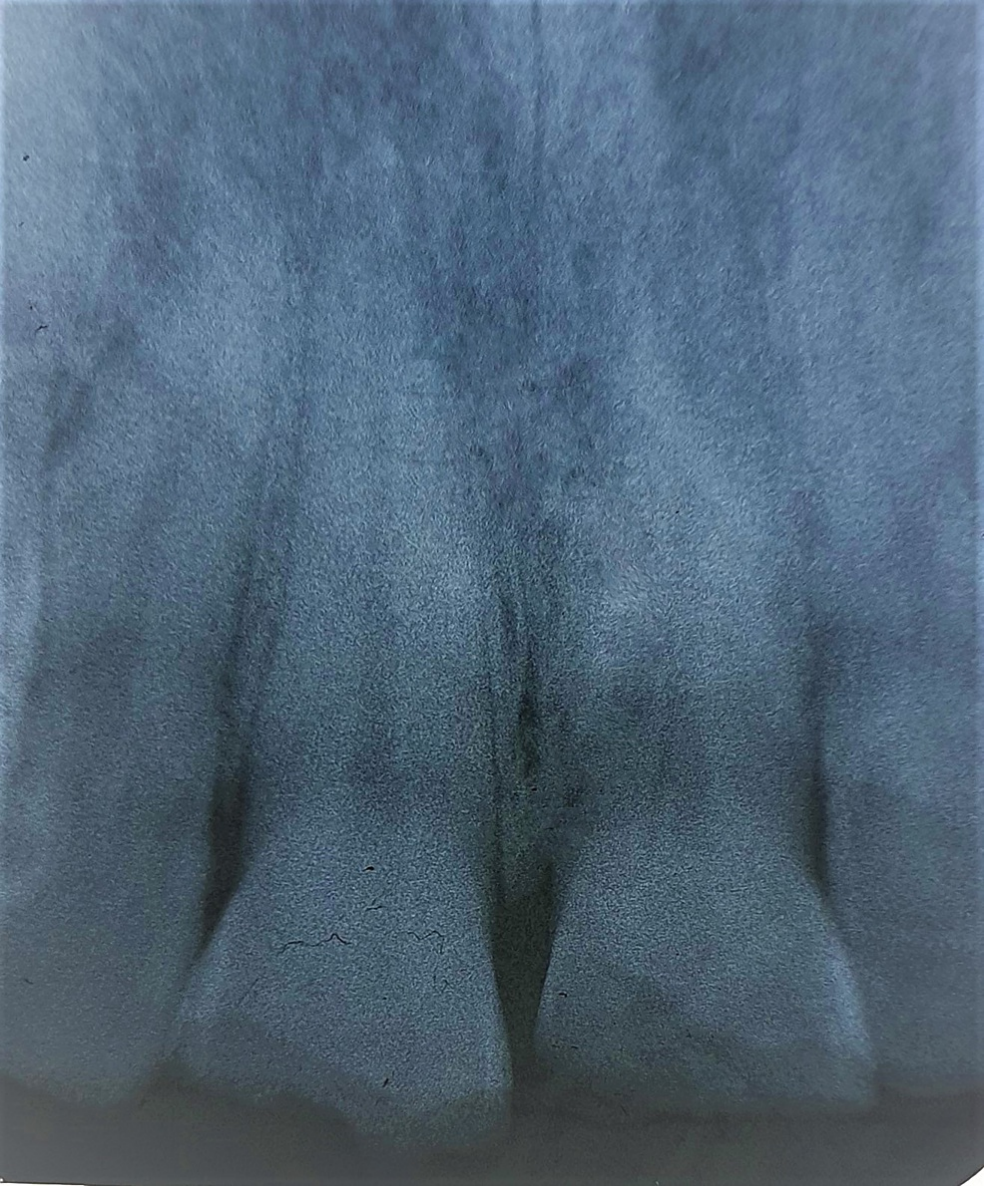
Radiographic examination validates the clinical observation and reveals the fracture line on teeth 11-21.
